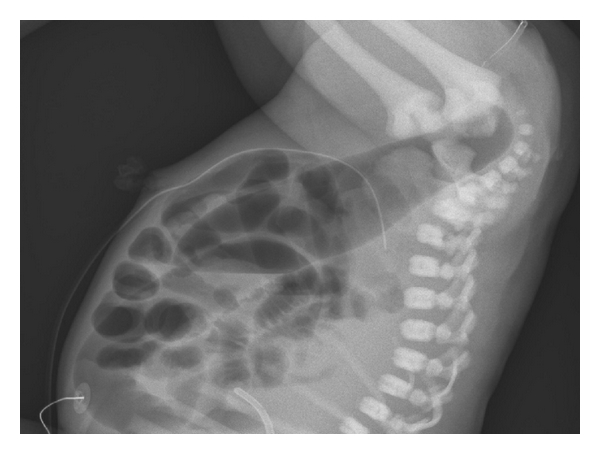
X-ray of abdomen at 24 hours of age before colostomy. A catheter is placed at the bottom of the anal channel.
